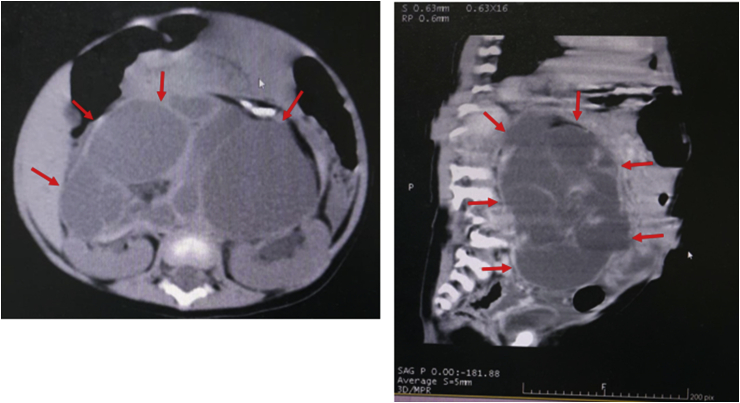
Abdominal CT scan revealed a complex cystic mass measuring approximately 11.4 × 10.9 × 7.3 cm and normal common bile duct (CBD).
